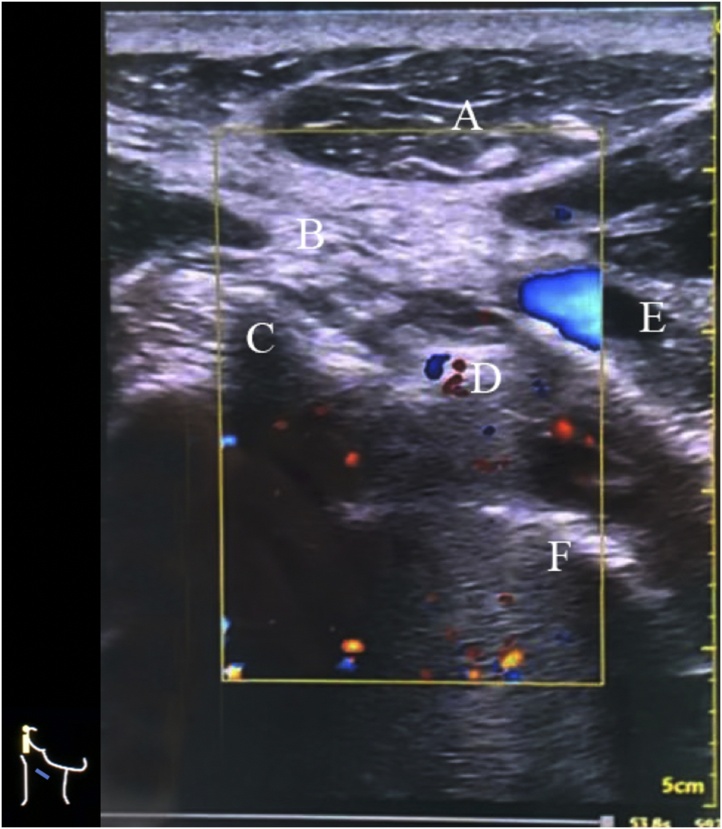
Ultrasound of the neck region. A: Sternocleidomastoid muscle; B: Needle’s track; C: Anterior tubercle; D: Air rifle bullet; E: Carotid artery; F: Vertebral body.
